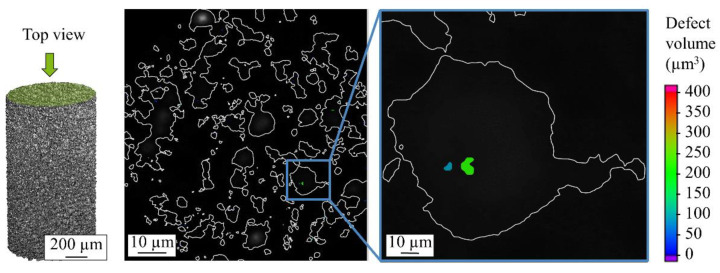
Top view 2D CT scan of the powder particles.
